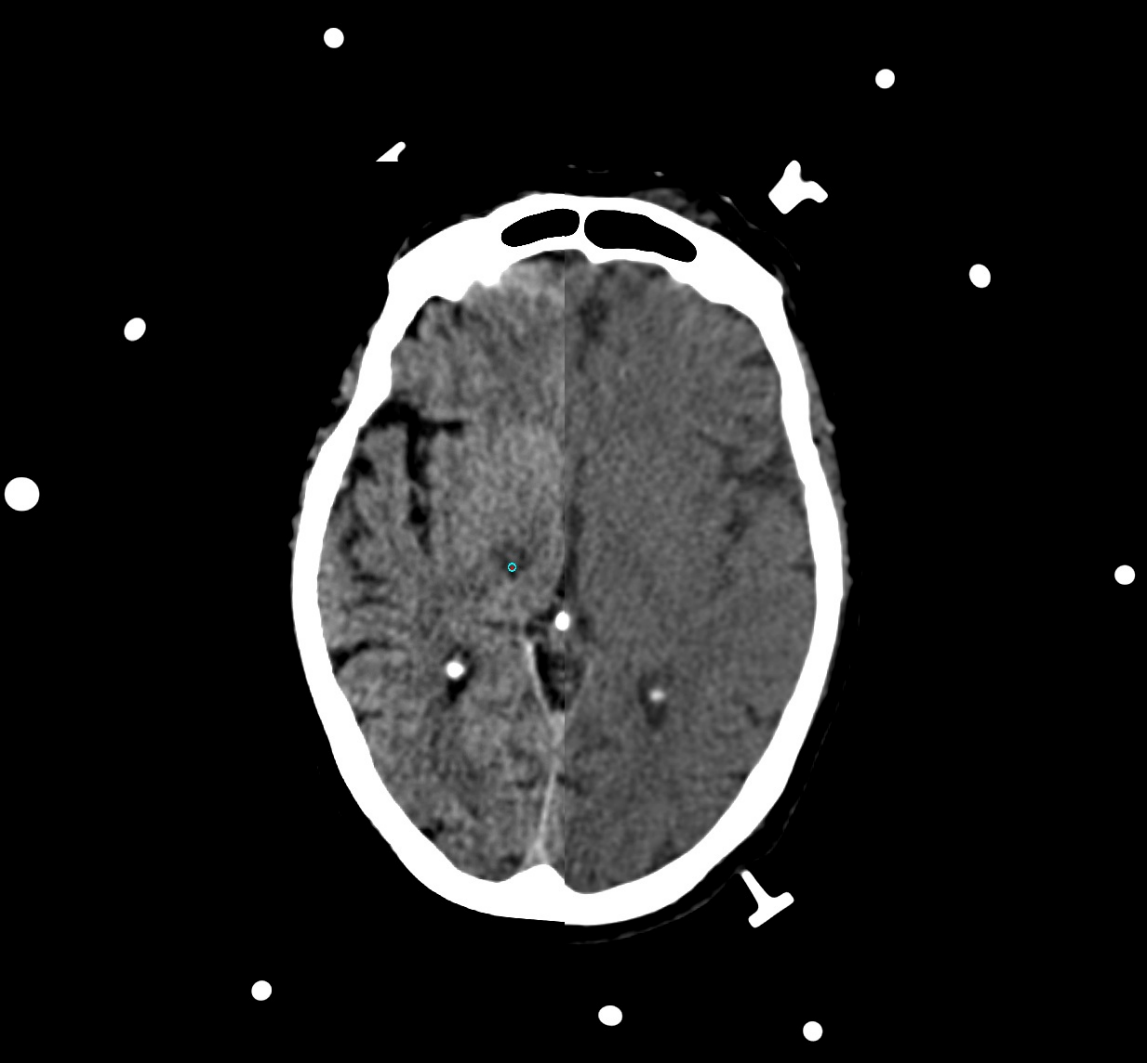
Thalamotomy at the target point The thalamotomy at the right ventro intermedius nucleus is visible as edema surrounding the target point depicted by the cyan circle in a post-operative, non-stereotactic computed tomography (CT) image (left) that is geometrically aligned to a pre-operative, stereotactic CT image (right).
